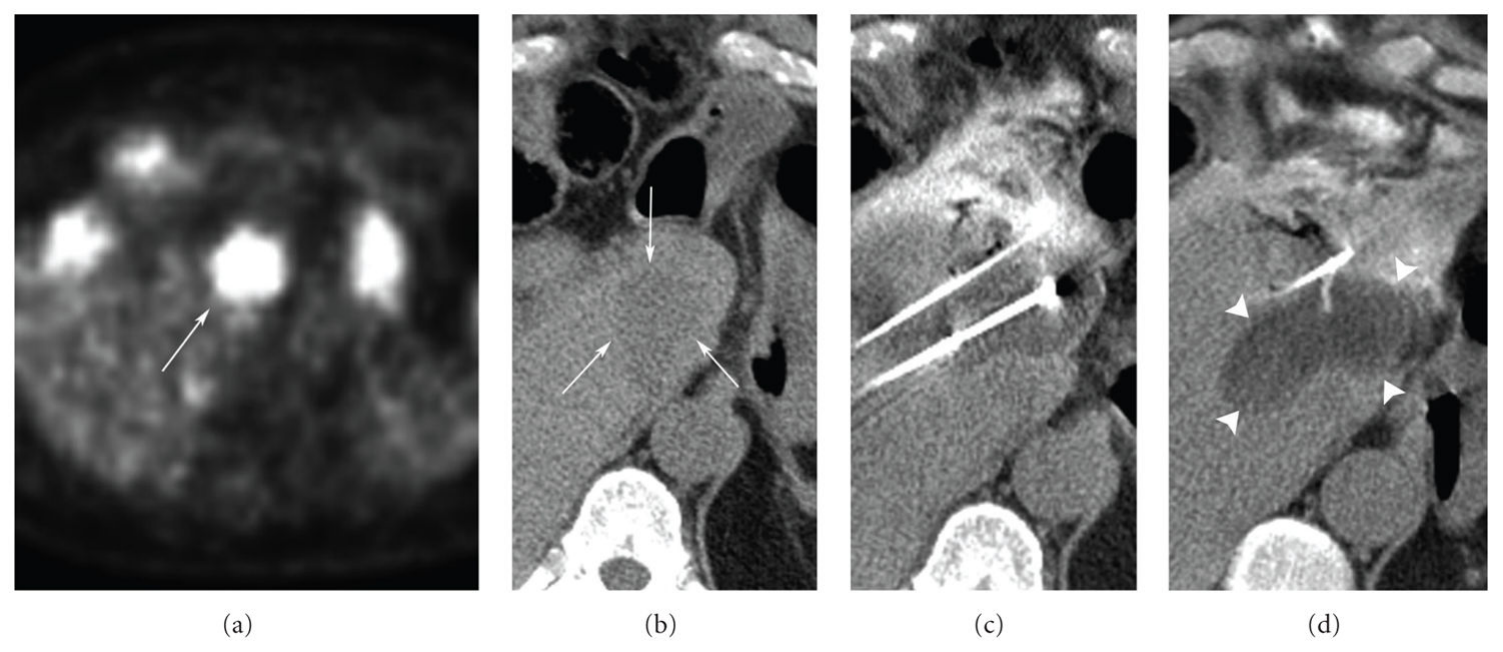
76-year-old male presenting with an FDG-PET positive recurrent lesion (a) from a cryoablation procedure 5 months prior. The caudate mass lies just anterior to the IVC and measures 3.3 × 3 × 3 cm (b). In order to avoid damaging the adjacent bowel, an 18-gauge Trocar needle was placed along the anterior/superior margin of the tumor, allowing for the injection of saline to provide hydrodissection protection. A total of three 2.4 mm cryoprobes bracketed the tumor, two of which abutted the IVC. Following a two, ten-minute freeze cycles ((c) and (d)), the ablation zone was visualized to extend beyond all tumor margins and produced final ice measurements of 4.2 × 5.5 × 5 cm. No new local recurrence was noted in subsequent follow-up imaging.
